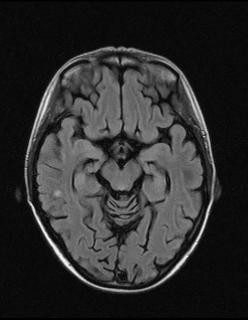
FLAIR image obtained at the patient's one-year follow-up magnetic resonance imaging (MRI) examination demonstrating the subcortical lesion at the level of the right temporooccipital white matter . The lesion was unchanged with regard to size and signal intensity compared with the previous MRI (see Figure 1A).
